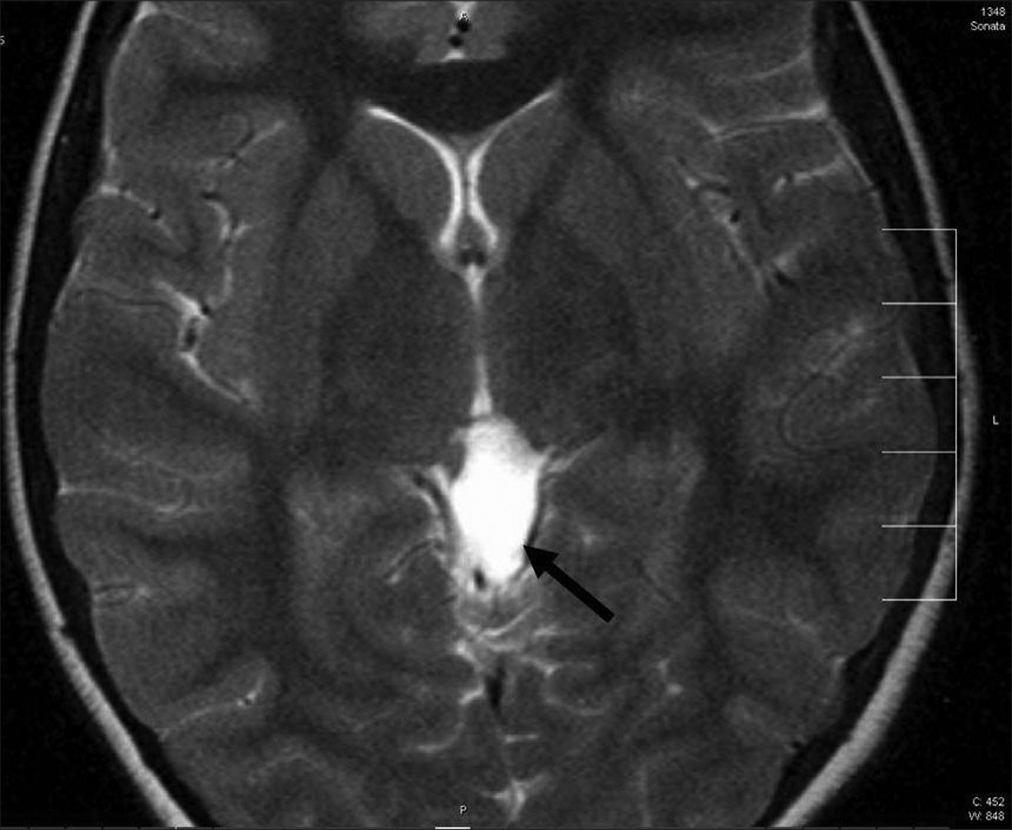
MRI brain of patient 1. T2-weighted image showing a mass in quadrigeminal cistern (arrow), isointense to cerebrospinal fluid, likely an arachnoid cyst, with compression of quadrigeminal plate
